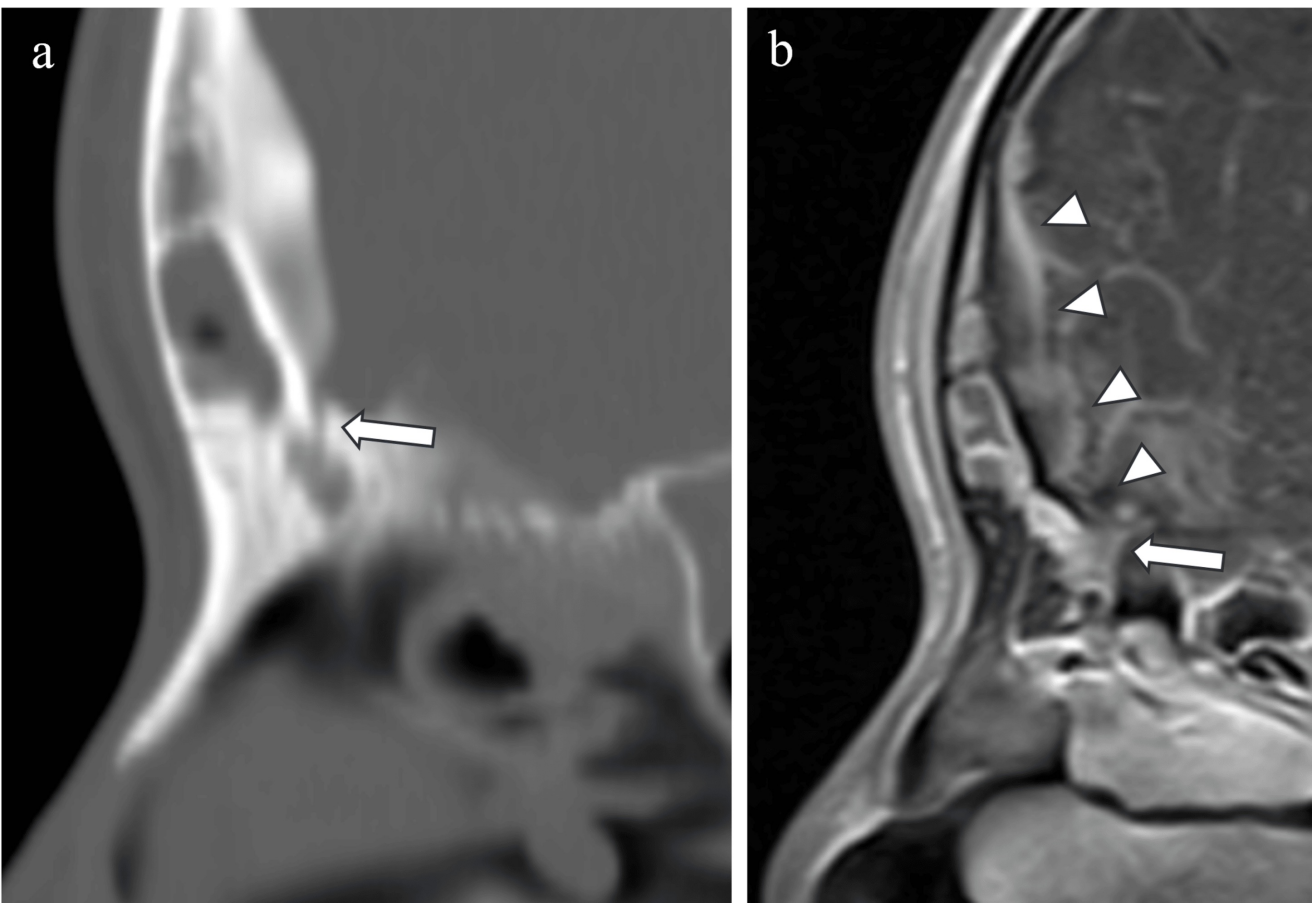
Sagittal Head Figures a) Sagittal CT, b) sagittal MRI The horizontal section shows a fissure from the right frontal sinus to the abscess area. Soft shadows were observed bilaterally in the maxillary, ethmoid, frontal, and sphenoid sinuses.
